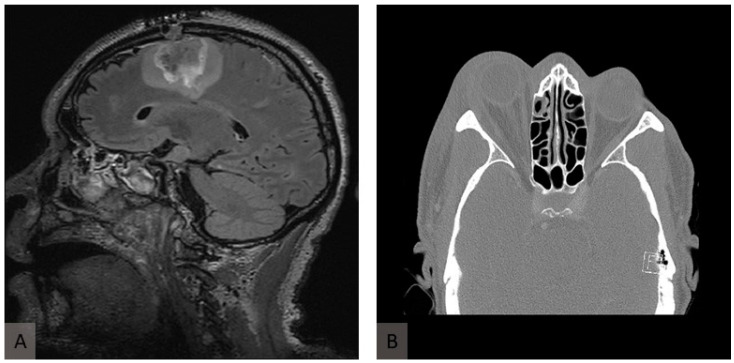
Radiological presentation of murocormycosis. (A) Axial fluid-attenuated inversion recovery (FLAIR) image of T2-weighted MRI of the head showing inflammatory changes in the frontal and parietal lobes in the sagittal plane. (B) A computed tomography scan at the initial presentation shows proptosis of the right eye and retrobulbar inflammation.
